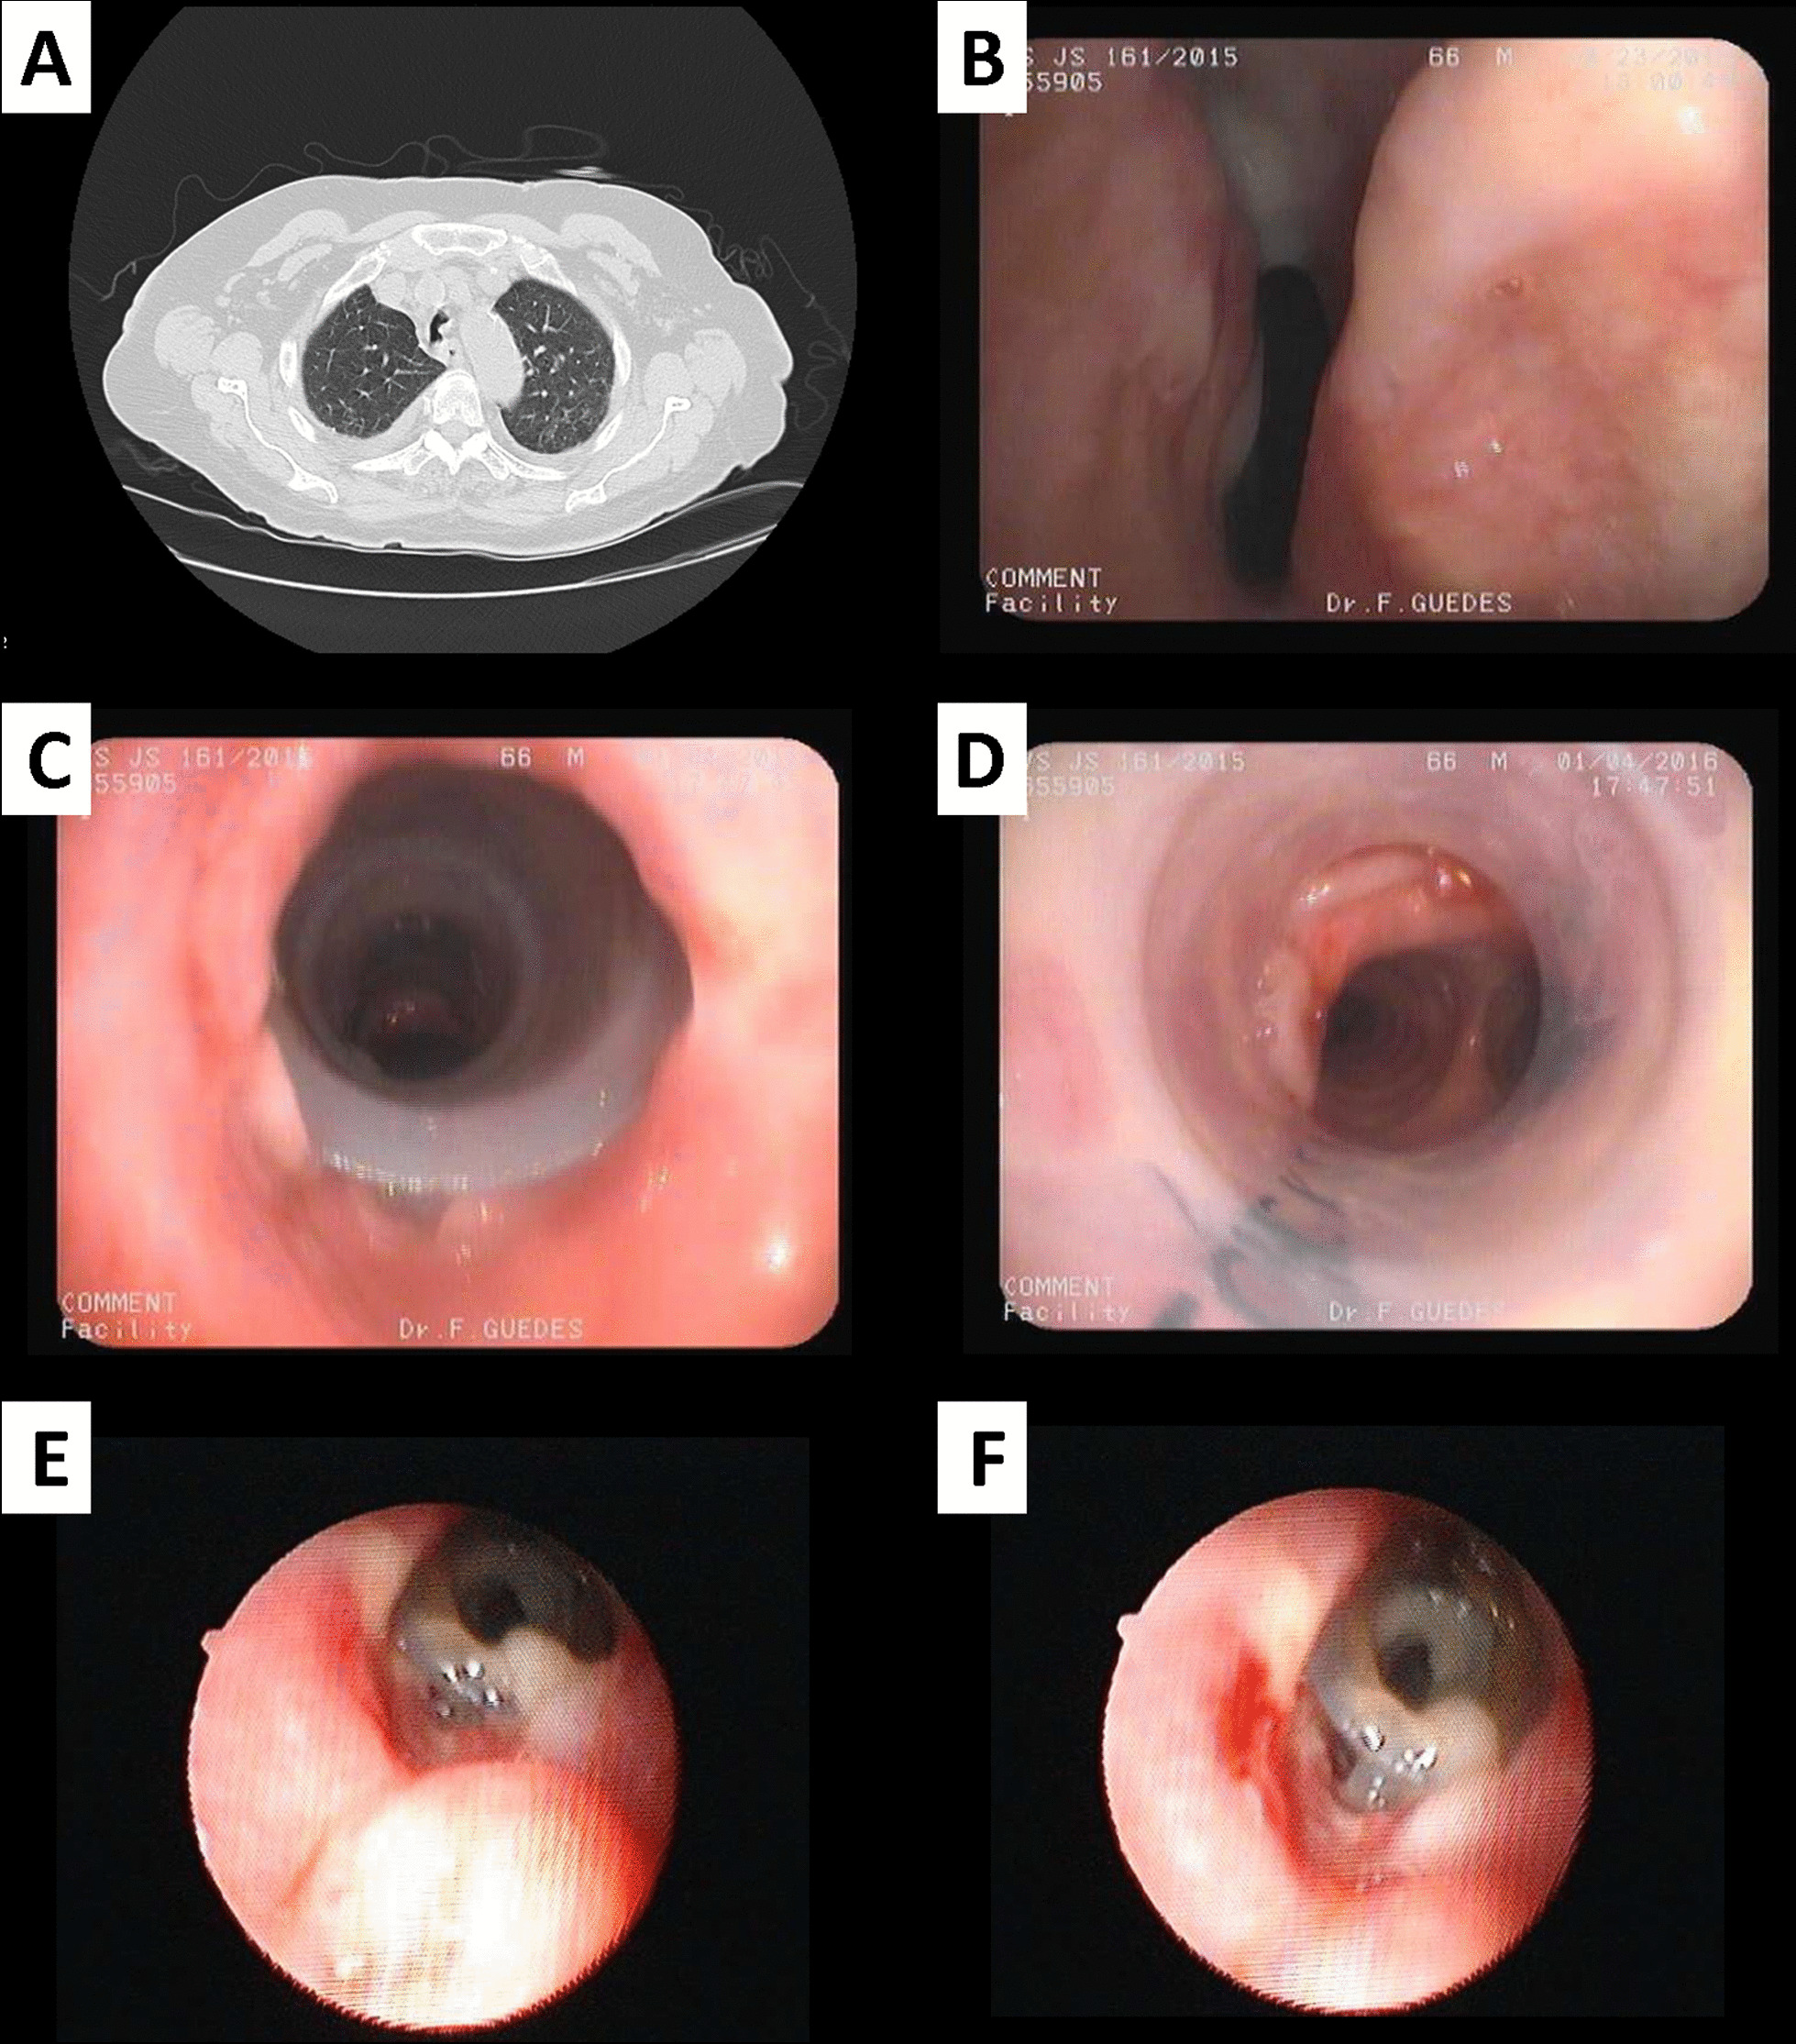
A CT scan shows a narrowing in the mid trachea; B Endoscopic vision of subglottic; C endoscopic view of trachea after placement of stent, showing the patency of airway; D Distal view of stent; E, F Stent migration and partially obstructed by mucus. E, F, Courtesy of Dr. José Almeida – Centro Hospitalar de Vila Nova de Gaia, Portugal
